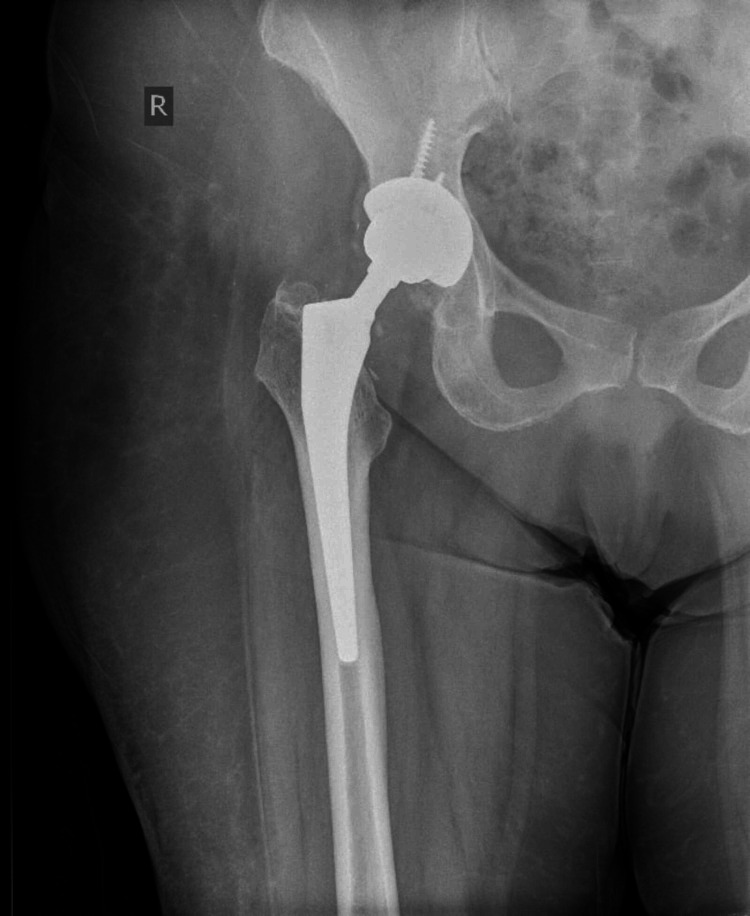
Postoperative hip radiograph after the revision of the ceramic bearings.
